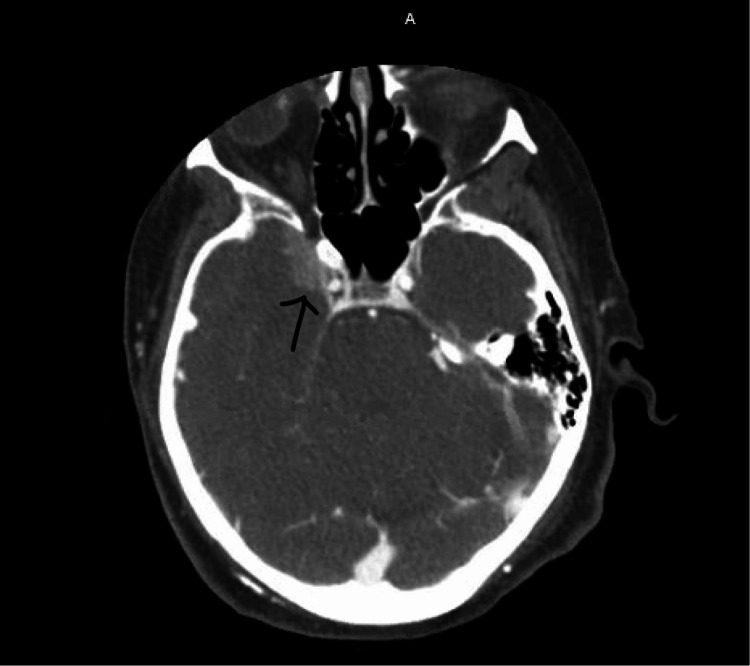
CT soft tissue head. The image demonstrates faint enhancement along medial right temporal lobe which could be reactive, infectious, low-grade glioma, meningioma, or artifact, measuring about 1.5 × 1.18 cm (arrow).
